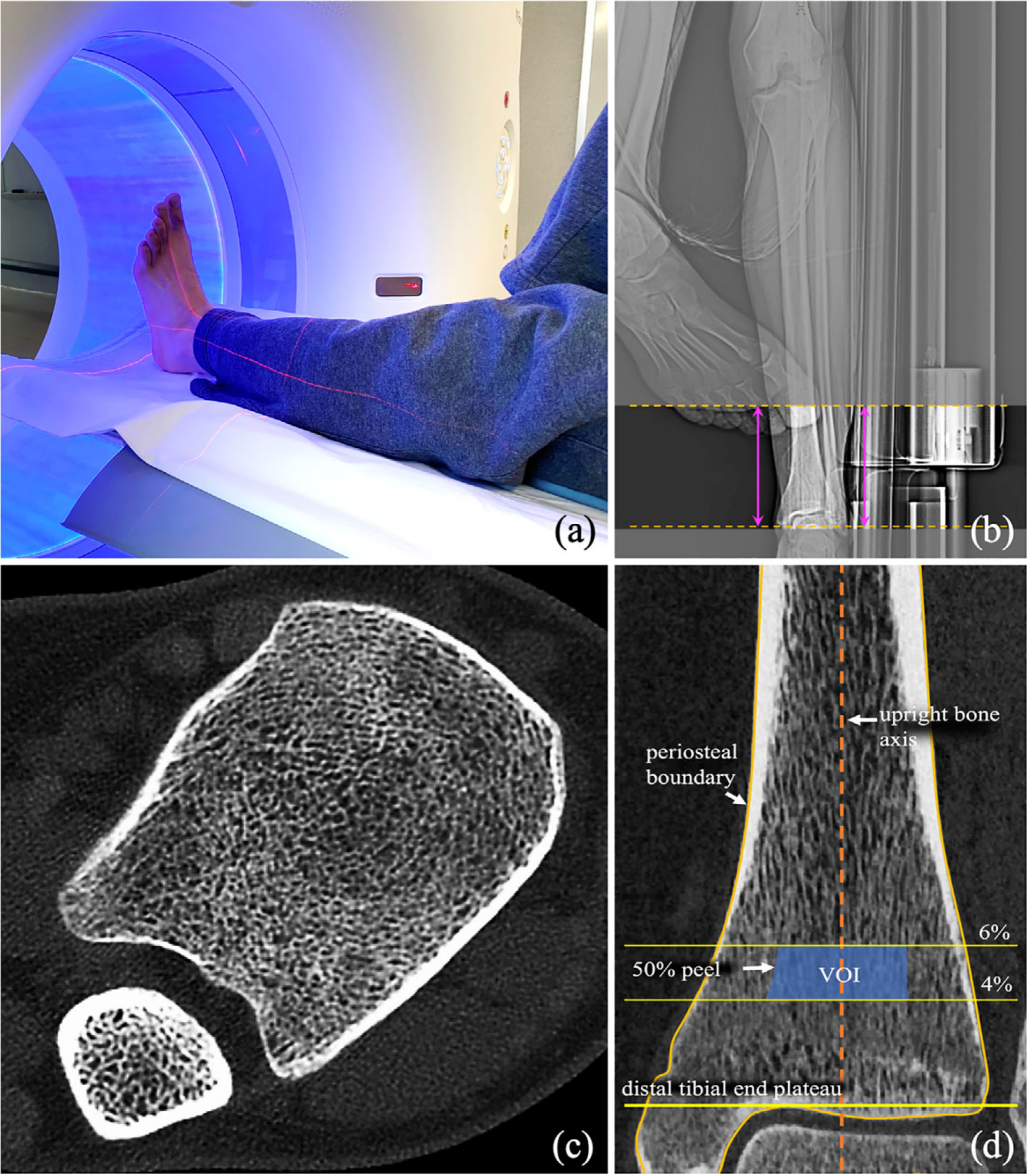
Setup for in vivo human ankle scan on a Siemens Flash CT scanner and VOI selection for FEA. (*A*) Positioning of the ankle after visually aligning the tibial axis with scanner center using laser rays to achieve maximum image resolution. (*B*) Position of the FOV on an anterior‐posterior projection CT scout scan including the distal tibial end plateau used to determine the VOI location for FEA. (*C*) An axial image slice from the reconstructed CT scan. (*D*) Location of the VOI on a sagittal image slice for FEA. FOV = field of view.
